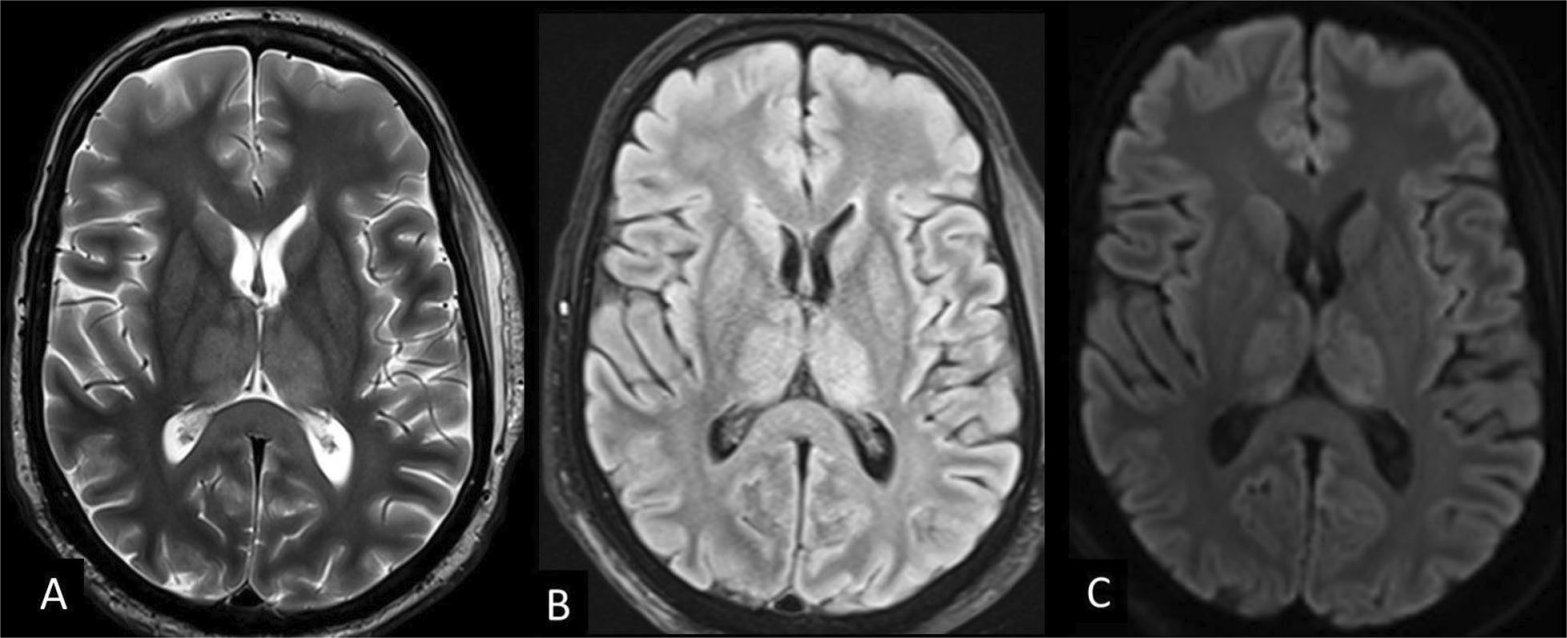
Hypoxic-ischemic encephalopathy. MRI images showing bilateral hypoxic-ischemic changes. a, b Axial T2-weighted and FLAIR images revealed in the basal ganglia and thalami. c DWI images show high diffusion signal in the basal ganglia and thalami
